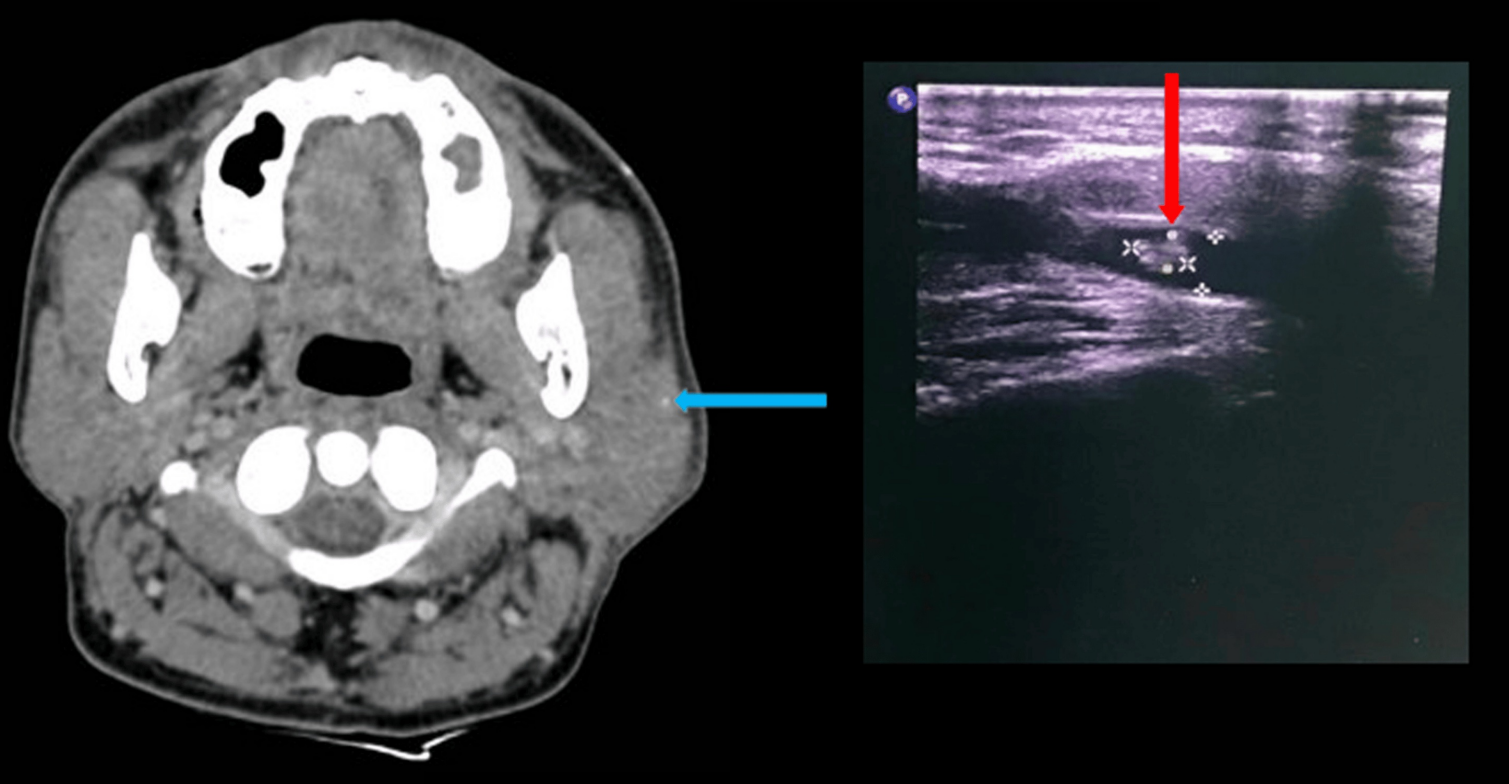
Ultrasound shows the left parotid salivary gland with a dilated duct (red arrow), and CT scan reveals the stone as a well-defined border area (green arrow)
